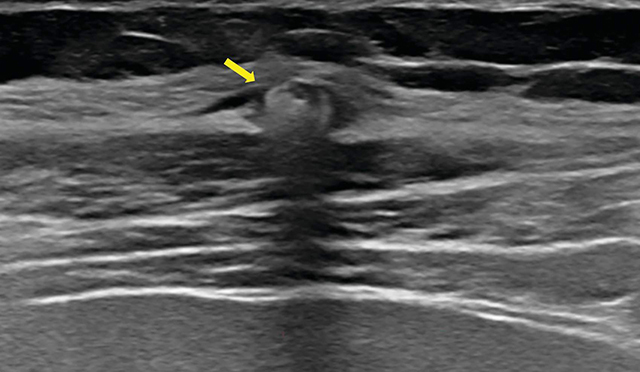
Ultrasound demonstrates some fluid accumulation and focal inflammation of the subcutaneous fat surrounding the affected electrode.
